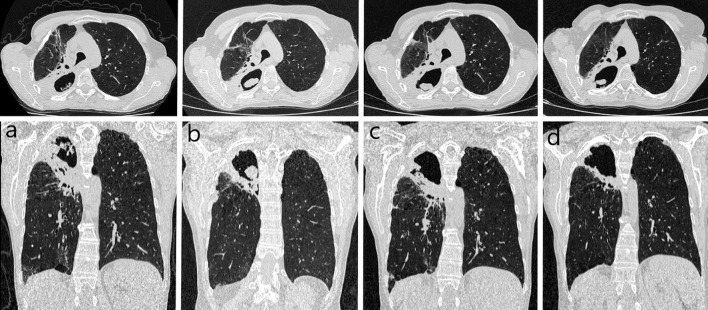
Controlled chest computed tomography during first-line treatment in 2020: (a) at the beginning of the therapy – irregular cavity size 102 x 59 mm in apical parts of the right lung, surrounded by solid consolidations widely adhere to the parietal pleura, connecting to the right hilum; (b) after 2 months – cavity with thick walls size 71 × 49 mm, concomitant mass on the posterior wall-size 29 x 10 mm; (c) after 4 months – cavity with thick walls size 69 × 47 mm, concomitant mass on the posterior wall-size 14 x 5 mm; (d) after 6 months – image stabilization.
